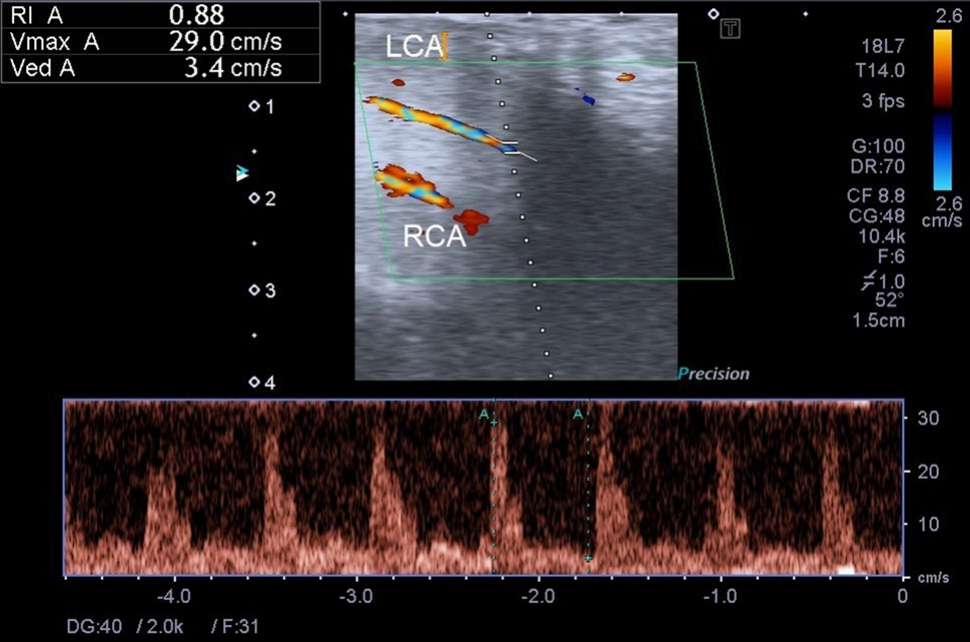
An example of dynamic duplex sonography of the penis. Both LCA and RCA are shown in this sonography. The hemodynamic parameters of the LCA are measured. The PSV (shown as Vmax A) is 29.0 cm/s, the EDV (shown as Ved A) is 3.4 cm/s, and the RI (shown as RI A) is 0.88. EDV: end-diastolic velocity, LCA: left cavernous artery, PSV: peak systolic velocity, RCA: right cavernous artery, RI: resistance index.
